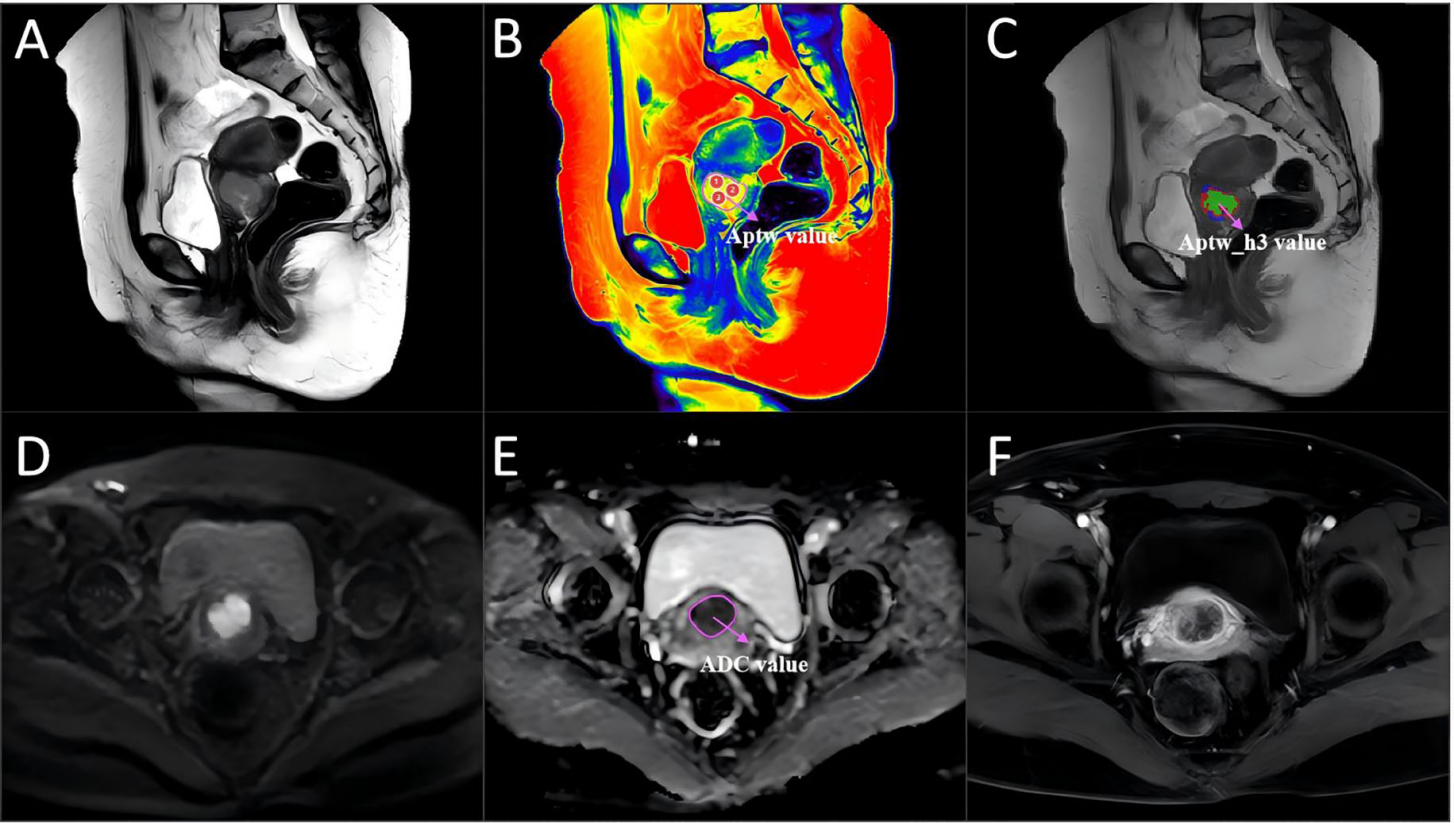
A 46-year-old woman with cervical cancer without LVSI, with a serum CA125 level of 16.8 U/mL and a largest tumor diameter of 31 mm. (A) Sagittal T2WI. (B) APTw image and T2W image fusion. (C) tumor region divided into three subregions via unsupervised clustering; red: subregion 1; blue: subregion 2; and green: subregion 3. (D) Diffusion-weighted image (b = 800 s/mm^2^). (E) ADC image. (F) DCE-MR image. The mean APTw and ADC values measured by the two radiologists were 2.31% and 1.74×10^−3^ mm^2^/sec, respectively. APTw, amide proton transfer-weighted; LVSI, lymphovascular space invasion.
